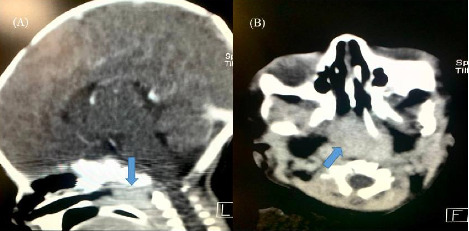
Spiral brain computed tomography (CT) scan. (A) Sagittal view and (B) axial (cranial) view. The arrows point to the enhanced adenoid region.
